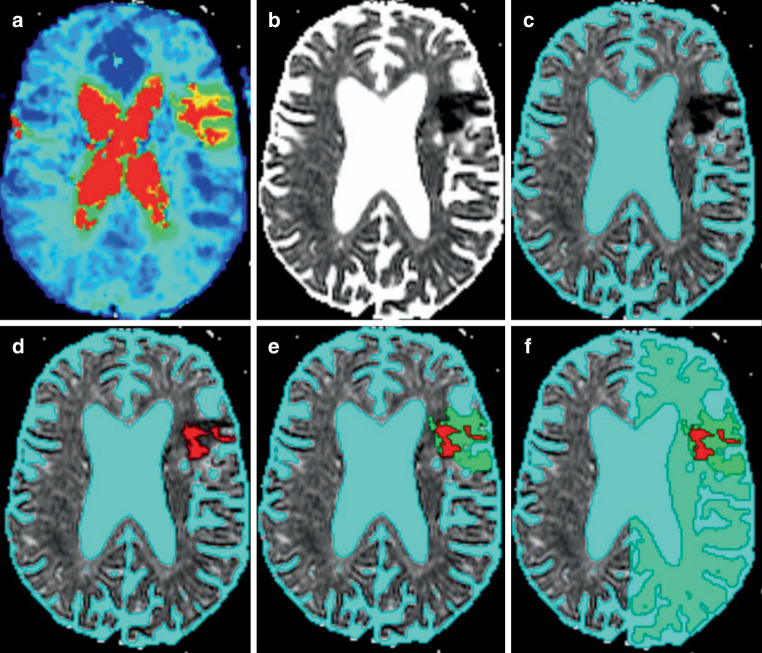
Axial section of the brain using multi-parametric MRI with semiautomatic imaging post-processing: a T-max map; b apparent diffussion (ADC) map; c ADC map with cerebrospinal fluid (CSF) segmentation; d infarct core region of interest (ROI) (*red*), e infarct core ROI (*red*) and penumbra ROI (*bright green*); f infarct core ROI (*red*), penumbra ROI (*bright green*), unaffected tissue ROI (*light green*)
